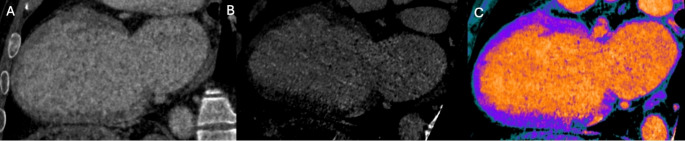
Dual-energy CT algorithm for myocardial tissue characterization. Panel A shows a faint area of late iodine enhancement (LIE) in the basal inferior segment with a non-ischemic distribution pattern on CT images acquired at 80 kV. Dual-energy reconstruction at low virtual monochromatic energy (55 keV) enhances iodine conspicuity, allowing clearer visualization of LIE (Panel B). The corresponding two-chamber iodine map (Panel C) further confirms the location and extent of iodine uptake
